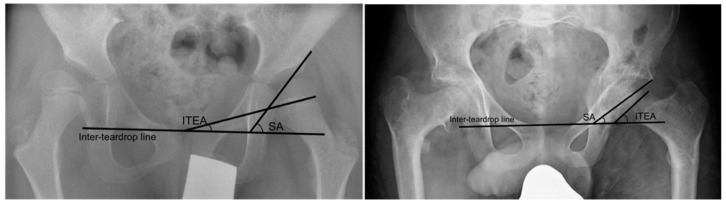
Pre-operative (left) and late post-operative (right) radiographs of a patient with bilateral CP who underwent bilateral hip reconstruction surgery.
